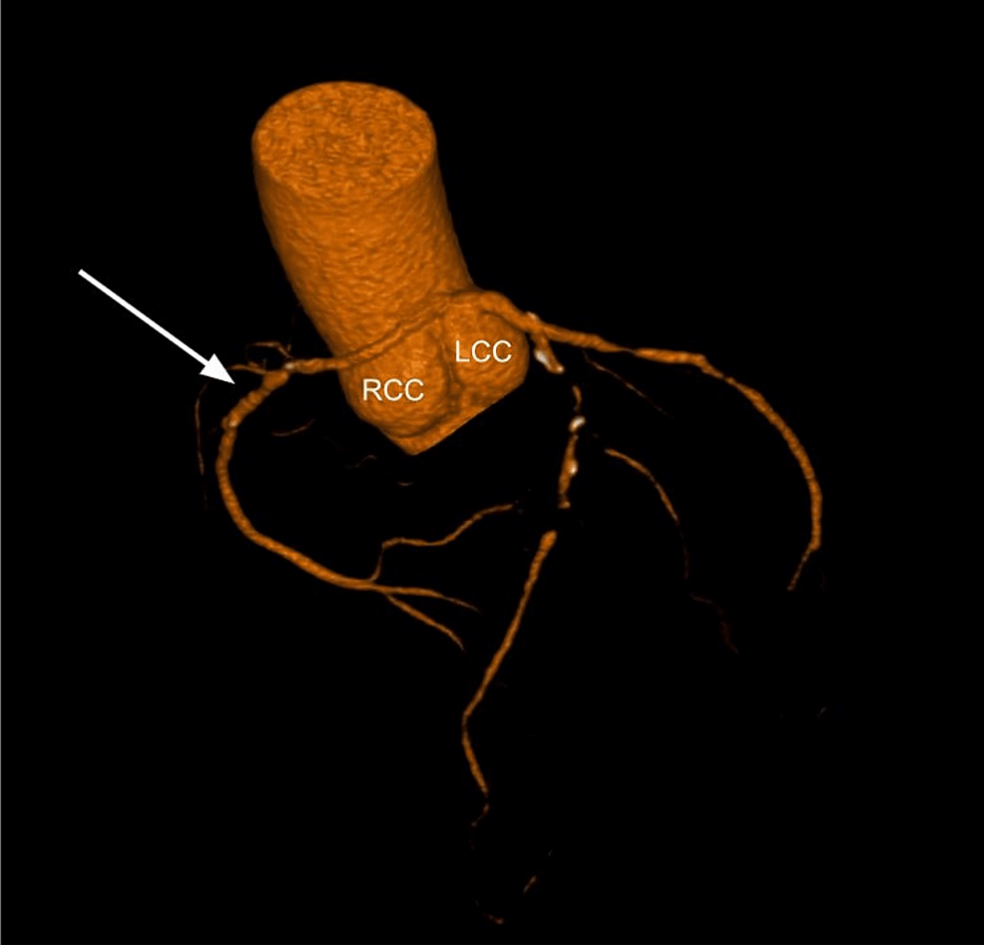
CTA coronary showing the anomalous origin of the RCA (arrow) arising from the left coronary cusp RCC: right coronary cusp; LCC: left coronary cusp; RCA: right coronary artery
